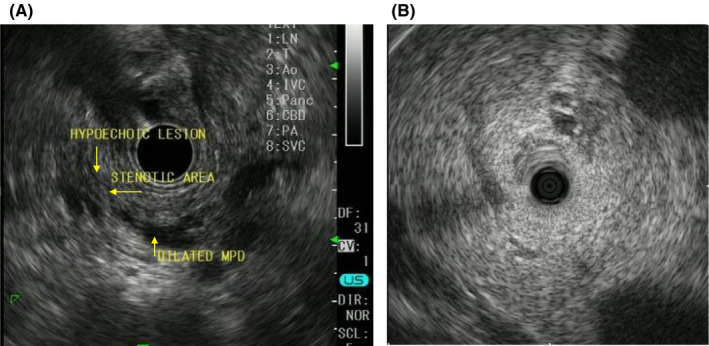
Endoscopic ultrasonography (A) and intraductal ultrasonography (B). The main pancreatic duct in the body and tail of the pancreas is dilated, but there is no visible tumor
